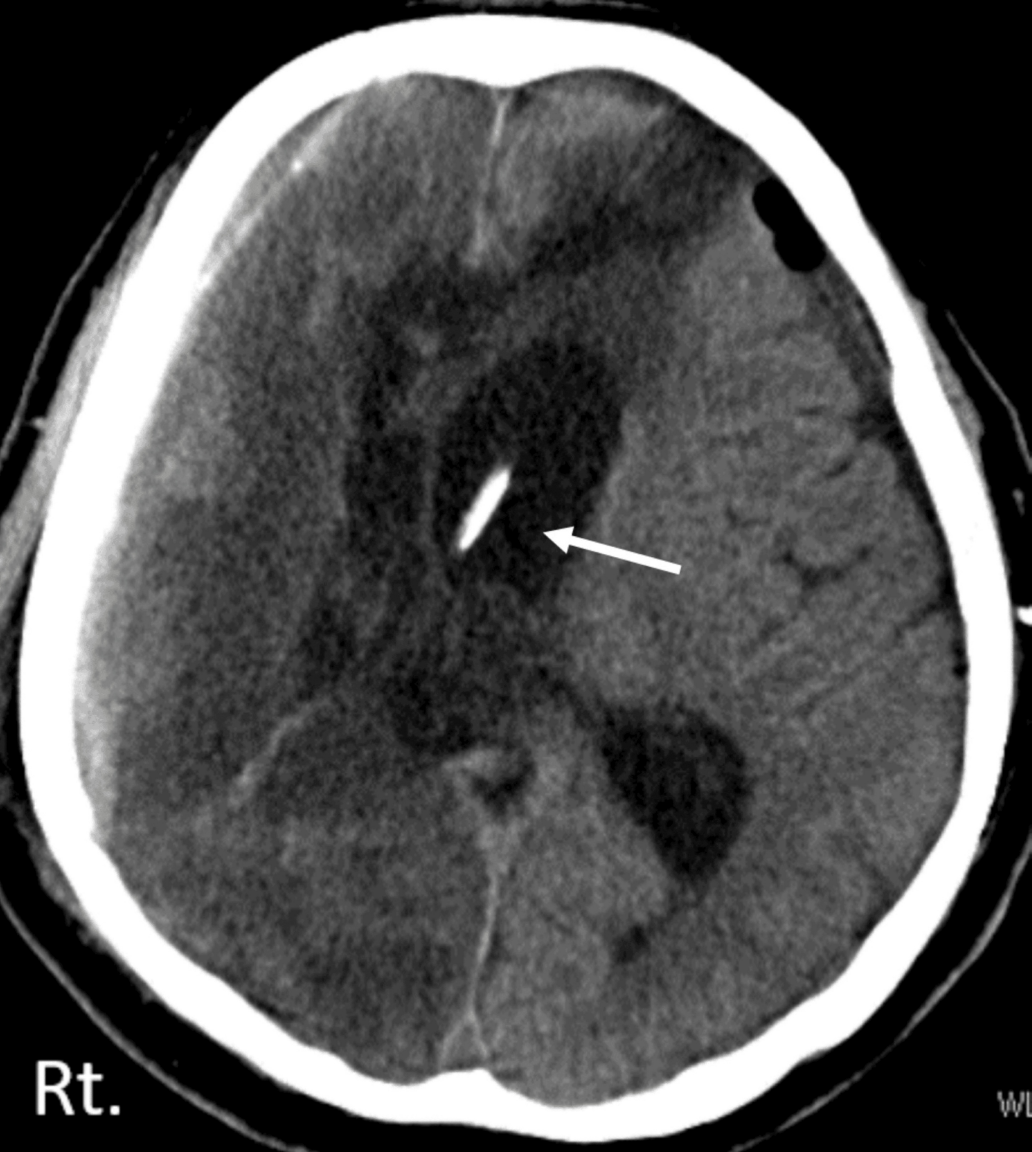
Head CT post-VP shunt surgery Head CT scan showed the tip of the ventricular shunt catheter in the left anterior horn (narrow white arrow).
